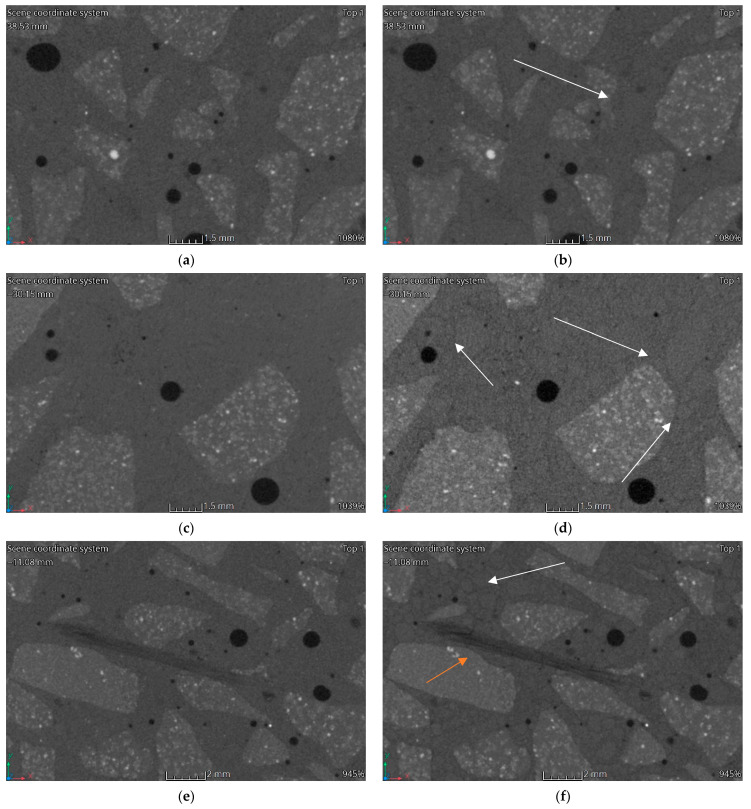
CT imaging results. Structural changes in three samples HPFRC 0.6: (a) 20 °C; (b) 200 °C; (c) 20 °C; (d) 400 °C; (e) 20 °C; (f) 600 °C.
